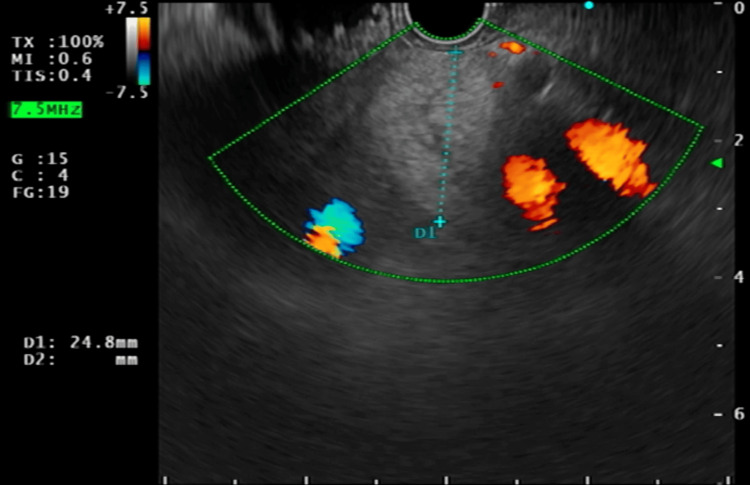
Endoscopic ultrasound image demonstrating a peri-pancreatic lymph node (24.8 mm) with colour Doppler flow
